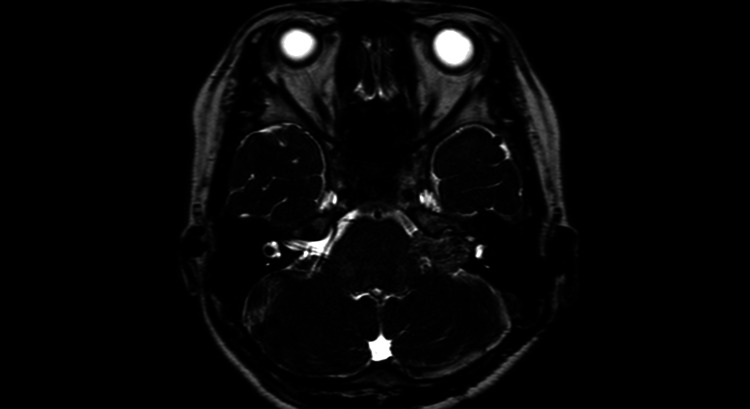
One year postoperative MRI heavy T2-weighted image
